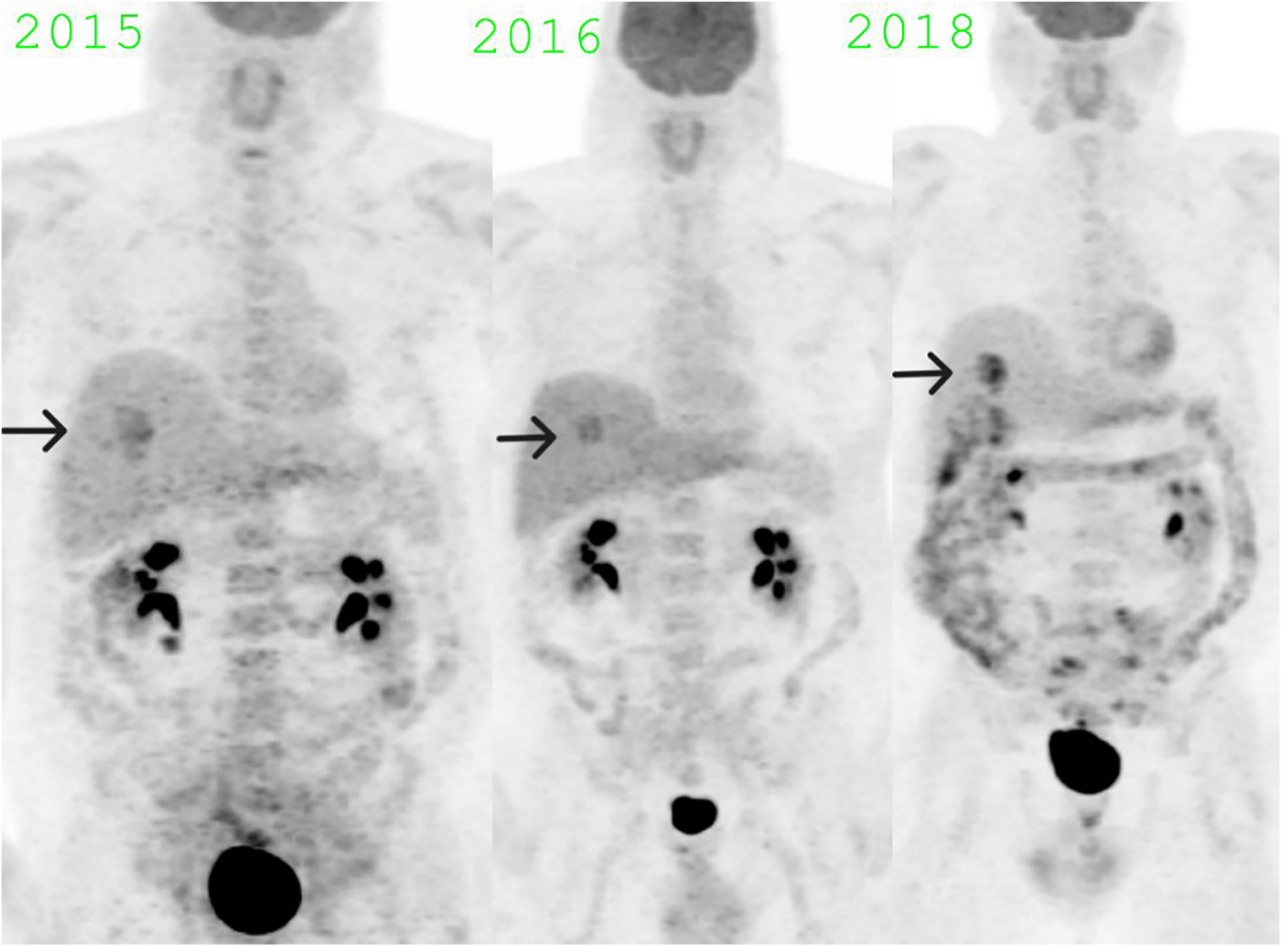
MIP images showing fluctuating SUV max in the hibernoma over the years: SUV max 6.5 in November 2015, SUV max 5.3 in June 2016, SUV max 7.8 in December 2018.
